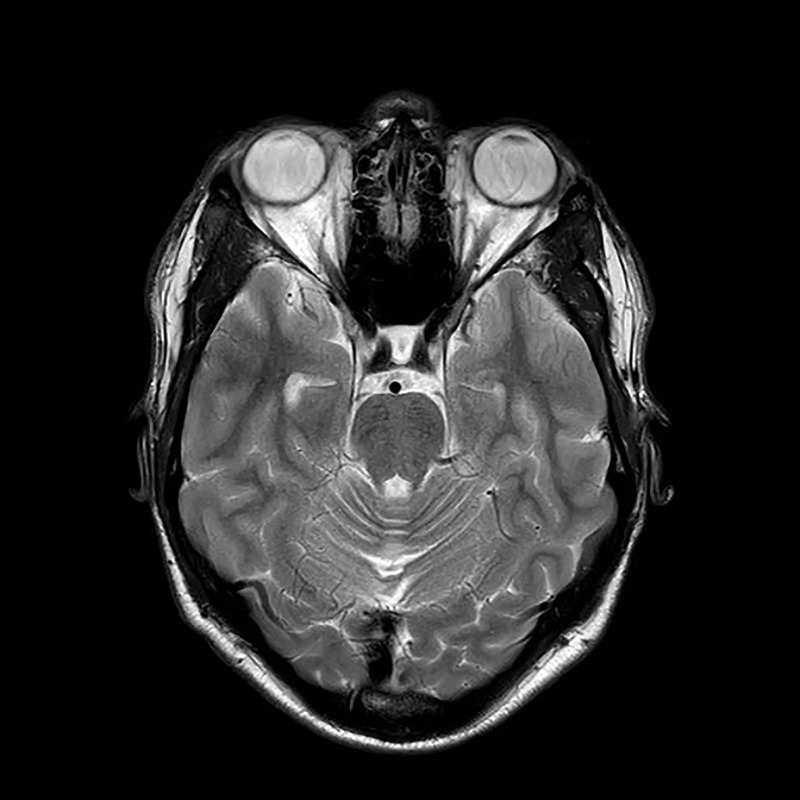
Axial T2-weighted MRI of the brain in a case of autosomal recessive spastic ataxia of Charlevoix-Saguenay (ARSACS) showing pontine hypointense (‘tigroid’) stripes.
